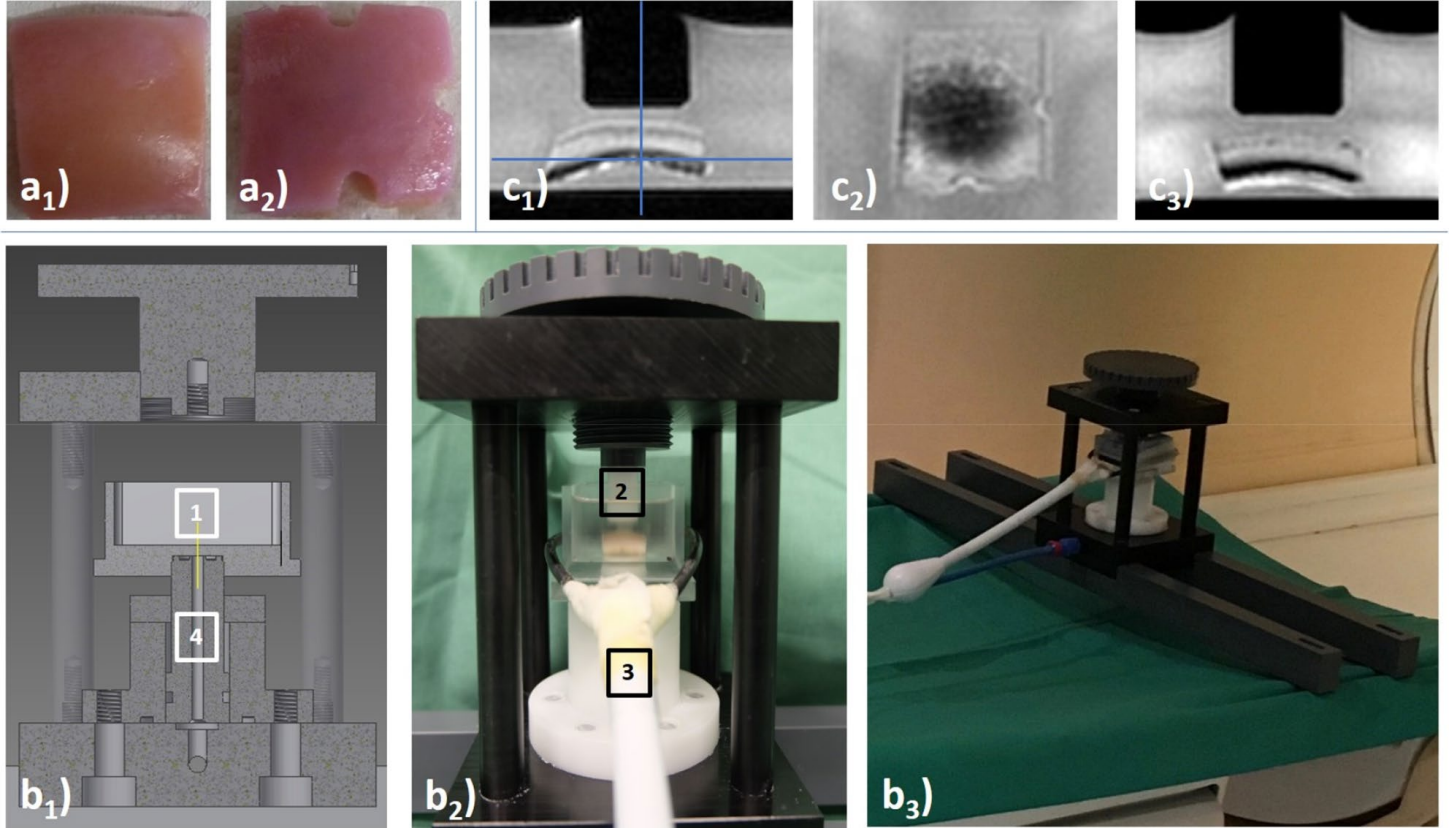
Details of cartilage preparation steps and experimental setup. (a) Top view of a representative osteochondral sample of standard square shape and size of 1.2 × 1.2 cm before (a_1_) and after (a_2_) creation of three notches to define the mid-sagittal imaging plane (extending from the 12 to the 6 o ‘clock position) and the sample centre (as the intersection of the mid-sagittal imaging plane and its orthogonal along the 3 o ‘clock position). (b) Cross-sectional construction sketch (b_1_) and photographs (lateral [b_2_] and angulated [b_3_] views) of the pressure-controlled indentation loading device outside (b_2_) and inside the MRI scanner (b_3_). Centrally positioned within the sample box (1), the cartilage sample was upwardly displaced against the indentor piston (2) that was adjustable in height. MR imaging was performed using a dedicated receiver coil that circumferentially comprised the sample box (3). Even though laid out to generate substantially greater forces, the pneumatic mechanism (4) was used to apply forces of 15.1 N and 28.6 N in the context of this study. For more details on the device, the interested reader is referred to Truhn et al., JMBBM, 2019^64^. (c) Sagittal (c_1_), axial (c_2_), and coronal (c_3_) Proton-Density-weighted images of a representative cartilage sample. Blue lines (in c_1_) indicate the respective heights of the axial (c_2_) and coronal (c_3_) images.
